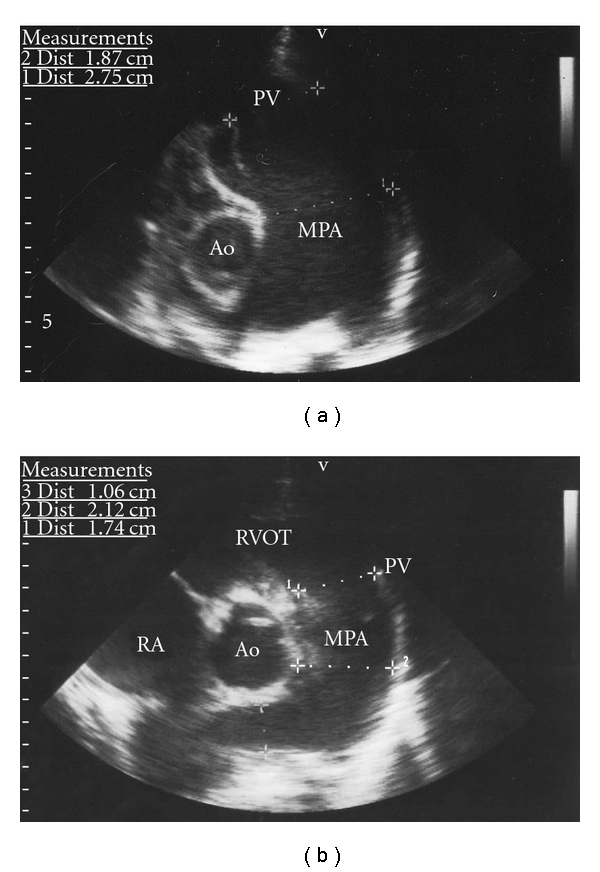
Parasternal short-axis view of the pulmonary valve (PV) and main pulmonary artery (MPA) of the patient preoperatively (a) and directly postoperatively (b). The severe dilatation of the PV (diameter 22 mm) and MPA (diameter 27 mm) diminished directly postoperatively, almost to normal limits (17.4 mm and 21.2 mm, resp.). RA: right atrium; RVOT: right ventricle outflow tract; Ao: aorta.
